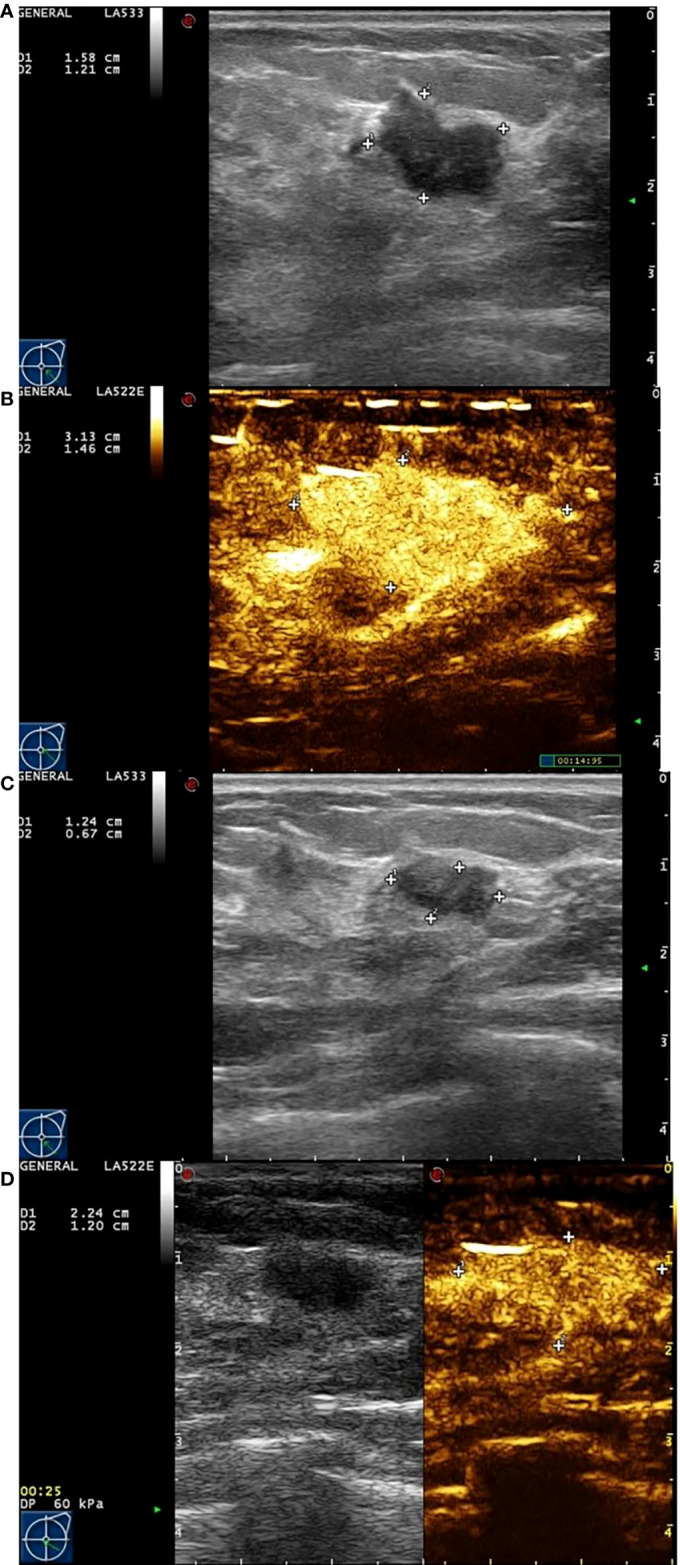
Images from a patient of non-response to NAC. (A) At baseline before NAC, the tumor size was measured by ultrasonography. (B) At baseline before NAC, the tumor size was measured by contrast-enhanced ultrasound. The extent of tumor was significantly larger than that of ultrasonography. (C) After the last cycle of NAC before surgery, the tumor size was measured by ultrasonography. (D) After the last cycle of NAC before surgery, the tumor size was measured by contrast-enhanced ultrasound. Although the extent of tumor has shrunken, there still have large number of contrast agents inside.
